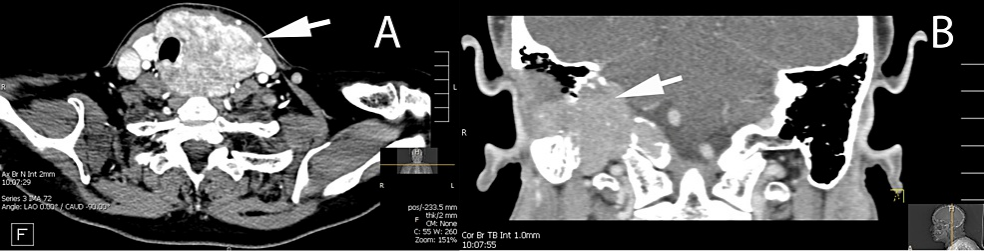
Computed tomography with contrast of neck and right skull base. (A) Avidly enhancing mass of the left thyroid gland (arrow) with tracheal deviation to the right. (B) Avidly enhancing mass centered in the right jugular foramen (arrow) with regional osseous destruction of the petrous and mastoid temporal bone.
